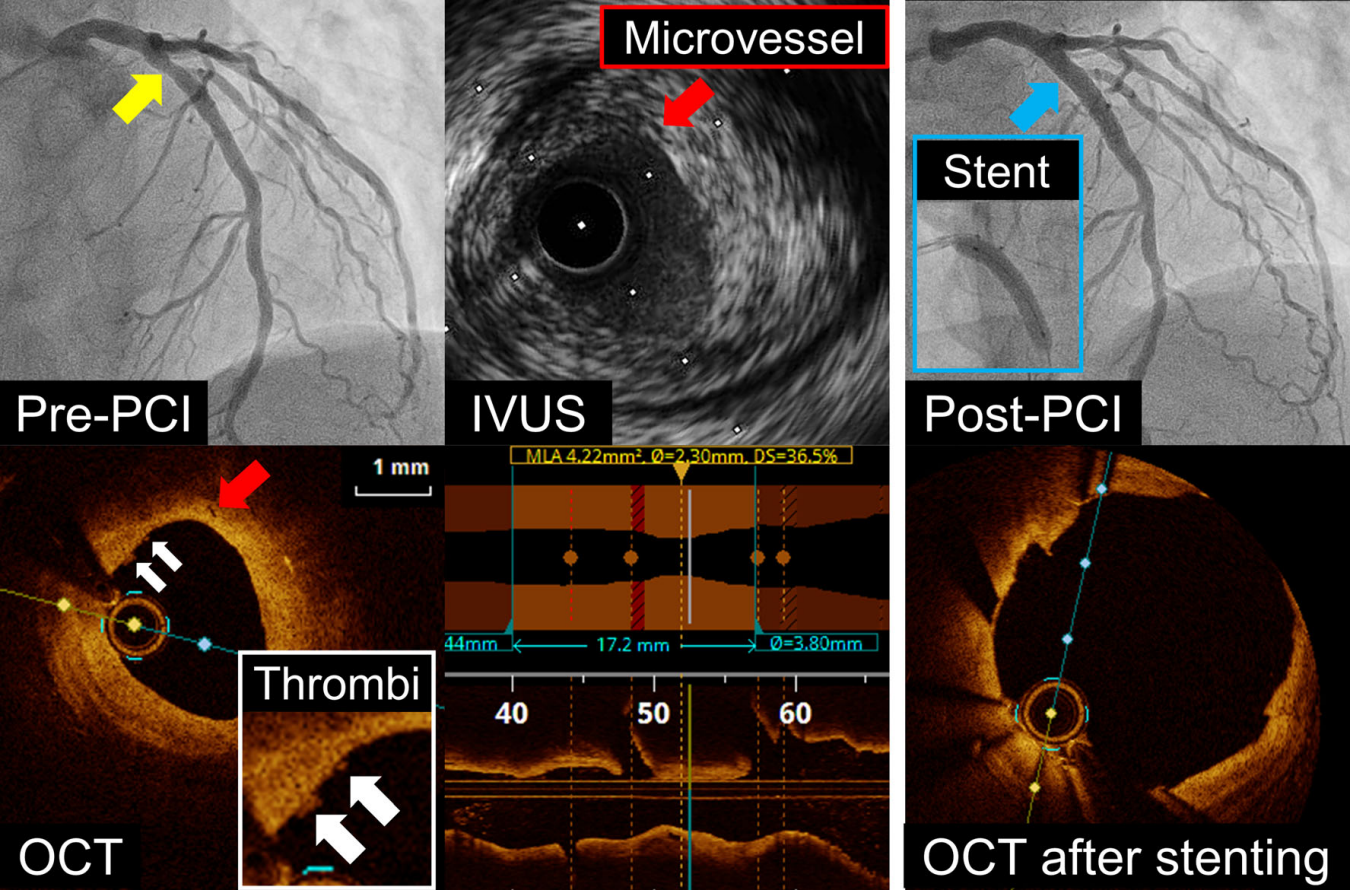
Invasive coronary angiography demonstrated a mild stenosis in the proximal left anterior descending coronary artery (yellow arrow). Intravascular ultrasound (IVUS) and optical coherence tomography (OCT) images showed coronary plaque with neovascularization (red arrows) and small thrombi (white arrows) at the minimum lumen area site, indicating the increased vulnerability of the coronary plaque. Percutaneous coronary intervention (PCI) with a drug-eluting stent (4.0 × 33 mm) was successfully performed (blue arrow)
